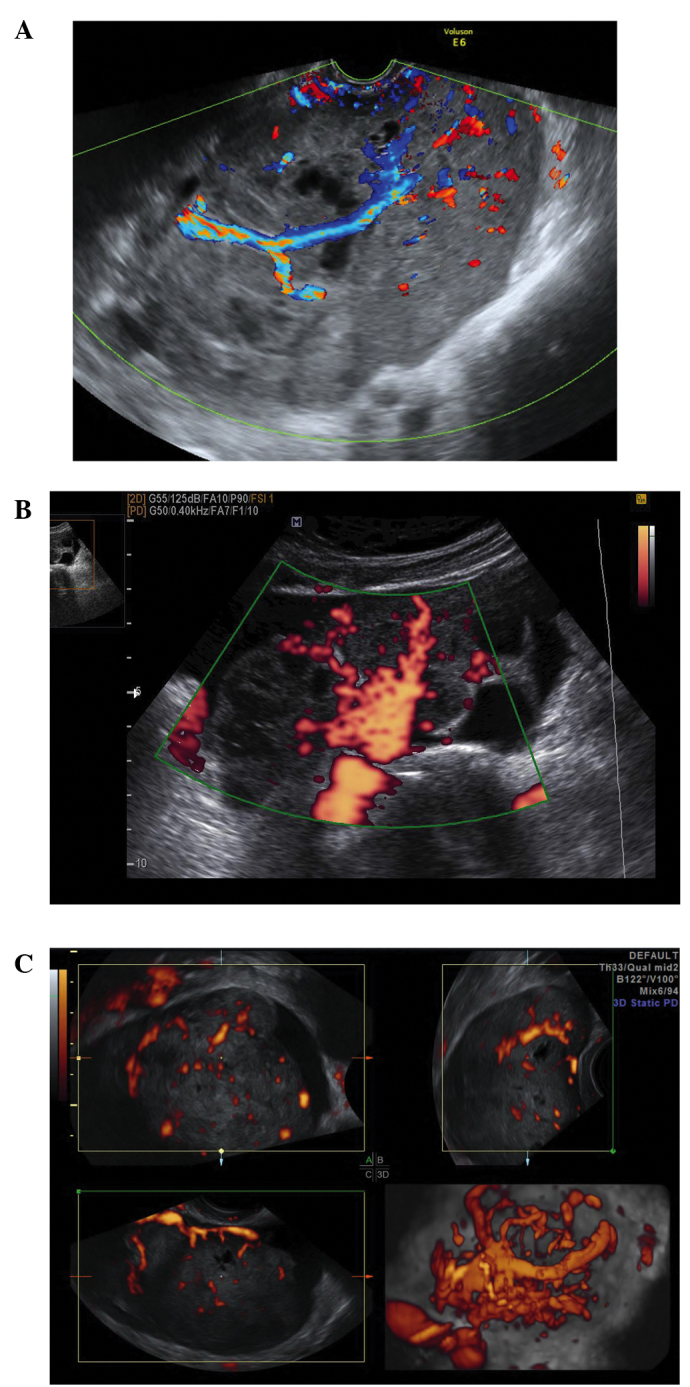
Breast cancer with metastasis to the ovaries. (A) Lead vessel sign in color Doppler 2D image. (B) Lead vessel sign in power Doppler 2D image. (C) Lead vessel sign in 3D power Doppler image.
